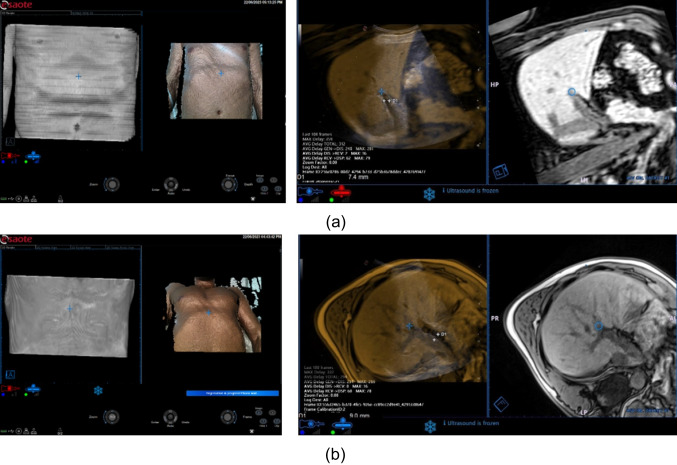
a Patient 1 MR images and skin surface acquired at 0-degree (left), (right) co-registration with an accuracy error of 7.4 mm, b Patient 2 MR image and skin surface 0-degree acquisition (left) co-registration result with accuracy error 9 mm (right)
